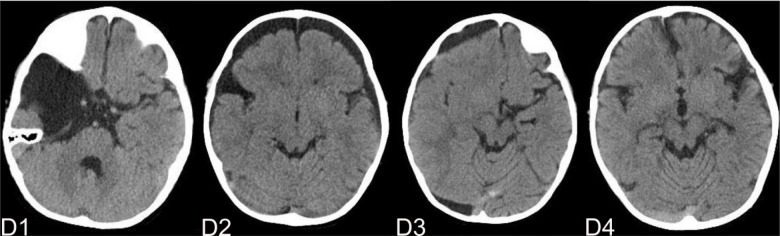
(D) shows the patient, who had subdural effusion after IAC operation during the follow-ups. D1 shows the CT image of IAC before operation. C2 shows the subdural effusion 3 month after operation. C3 shows the subdural effusion which decreases after the surgical treatment. C4 shows the disappearance of the subdural effusion 6 months latter.
